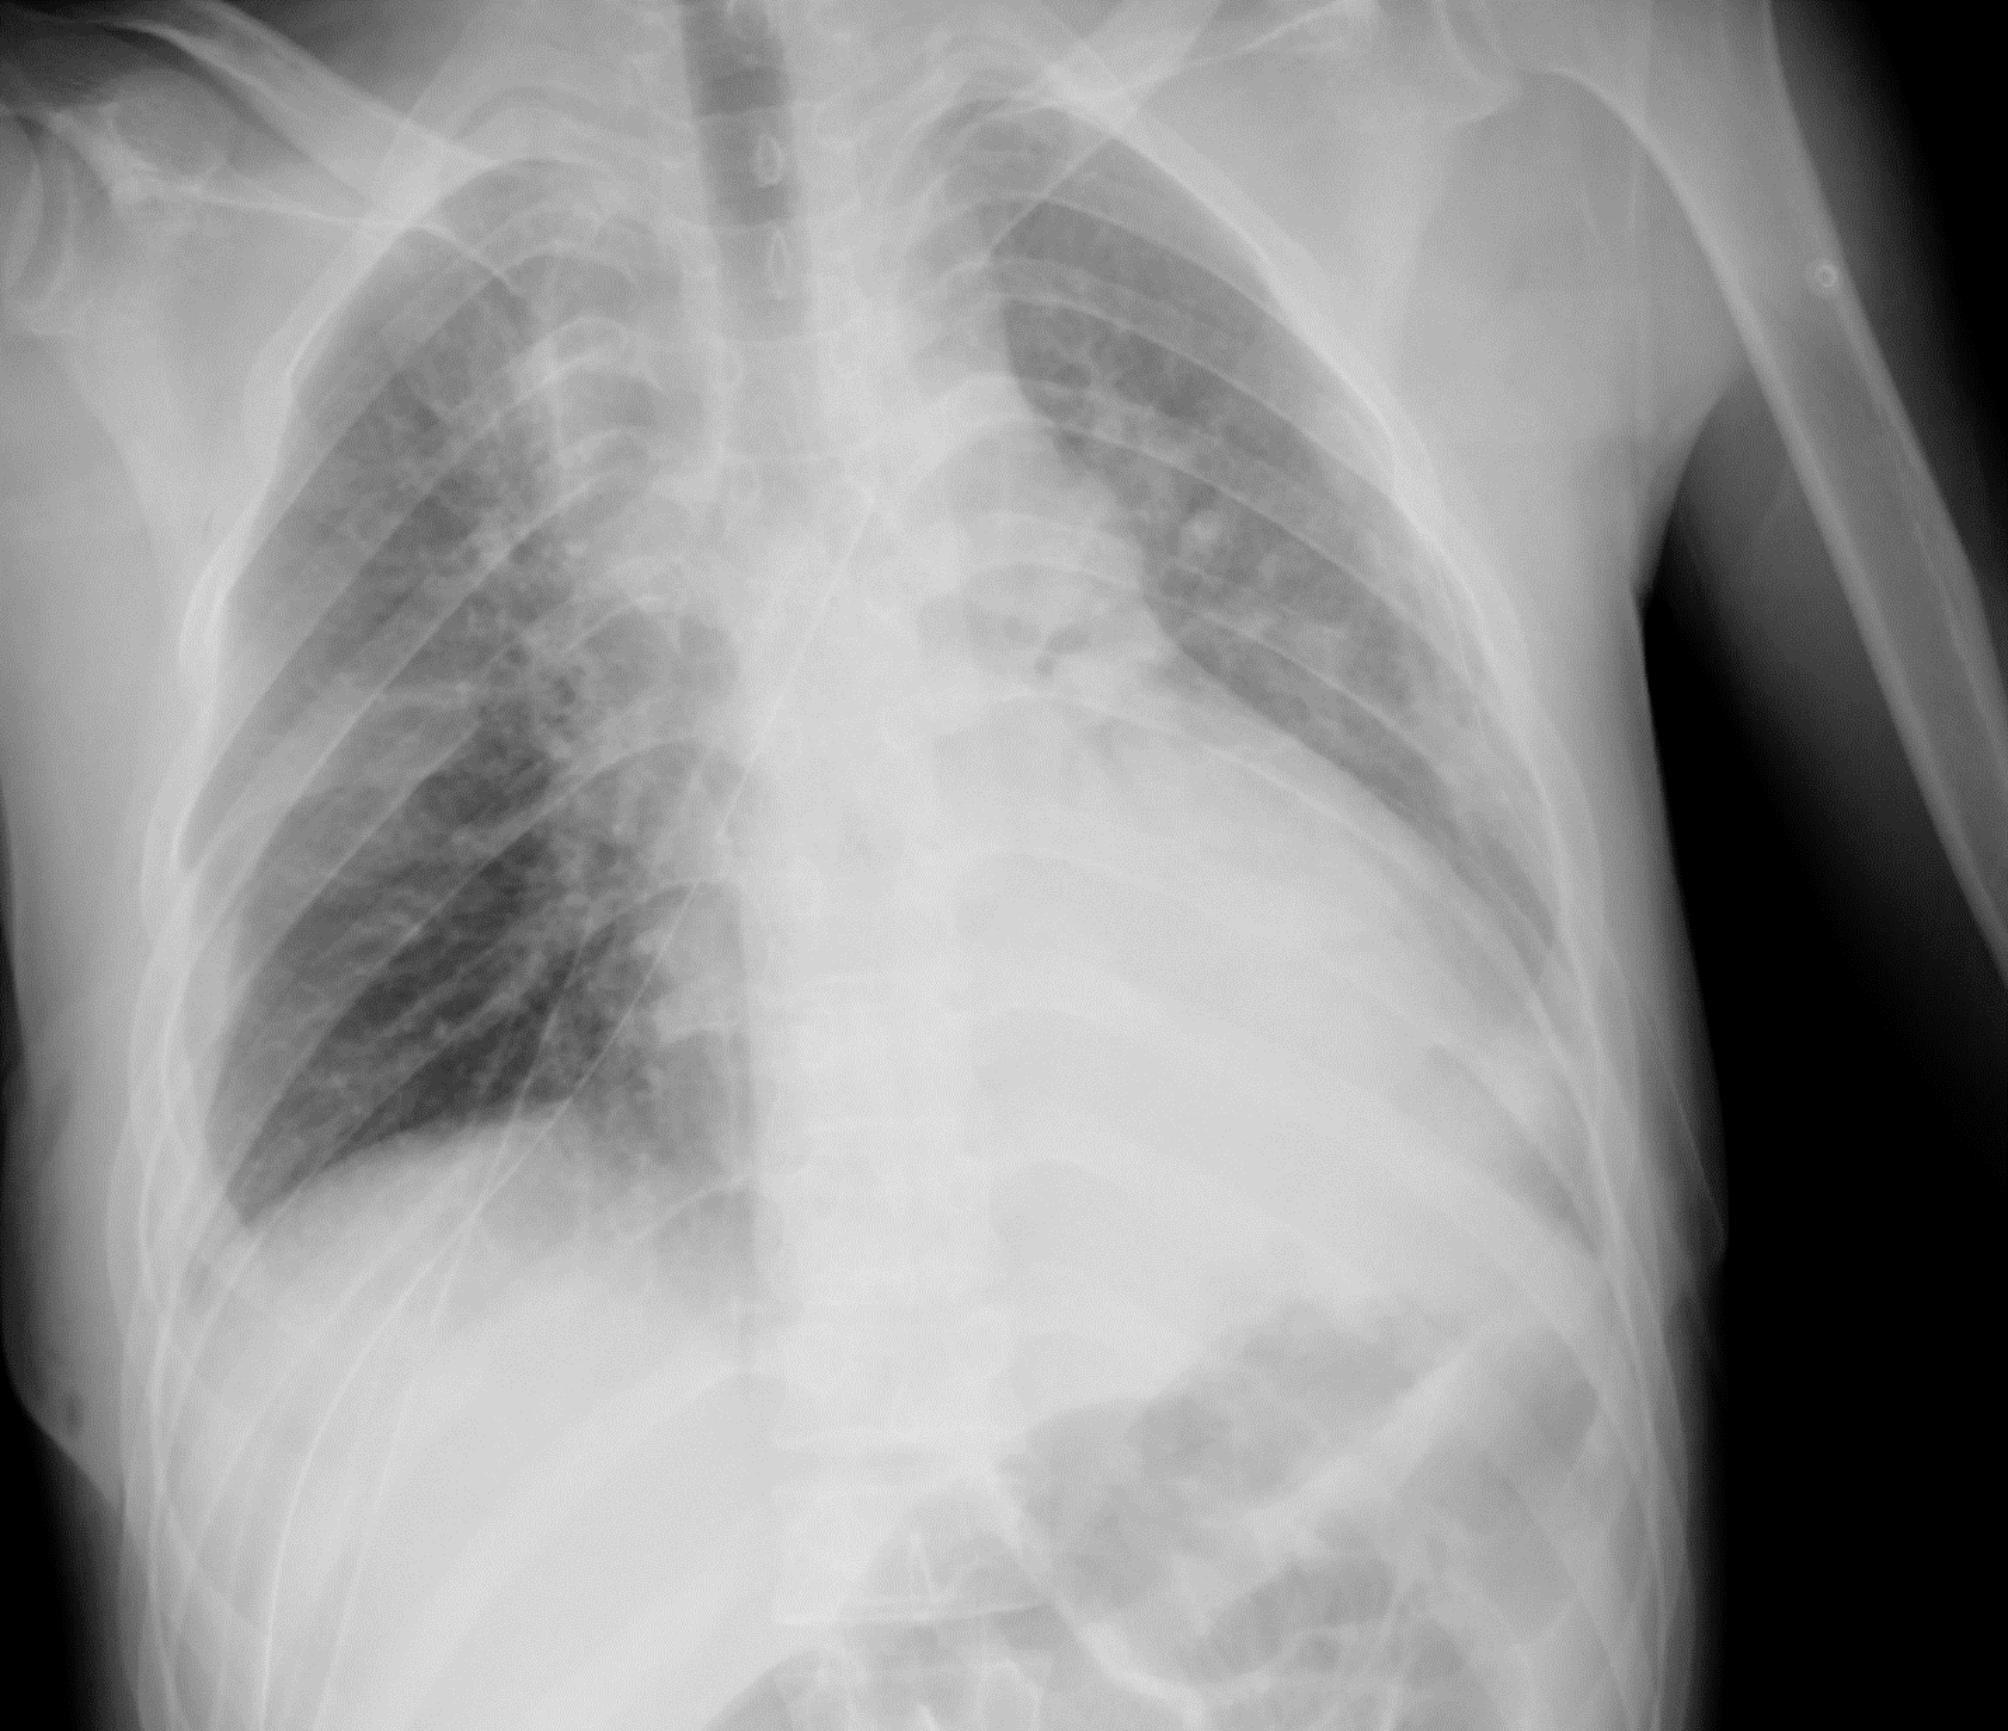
Postoperative chest x-ray after thoracotomy due to large mediastinal teratoma (4 days after the surgery)
